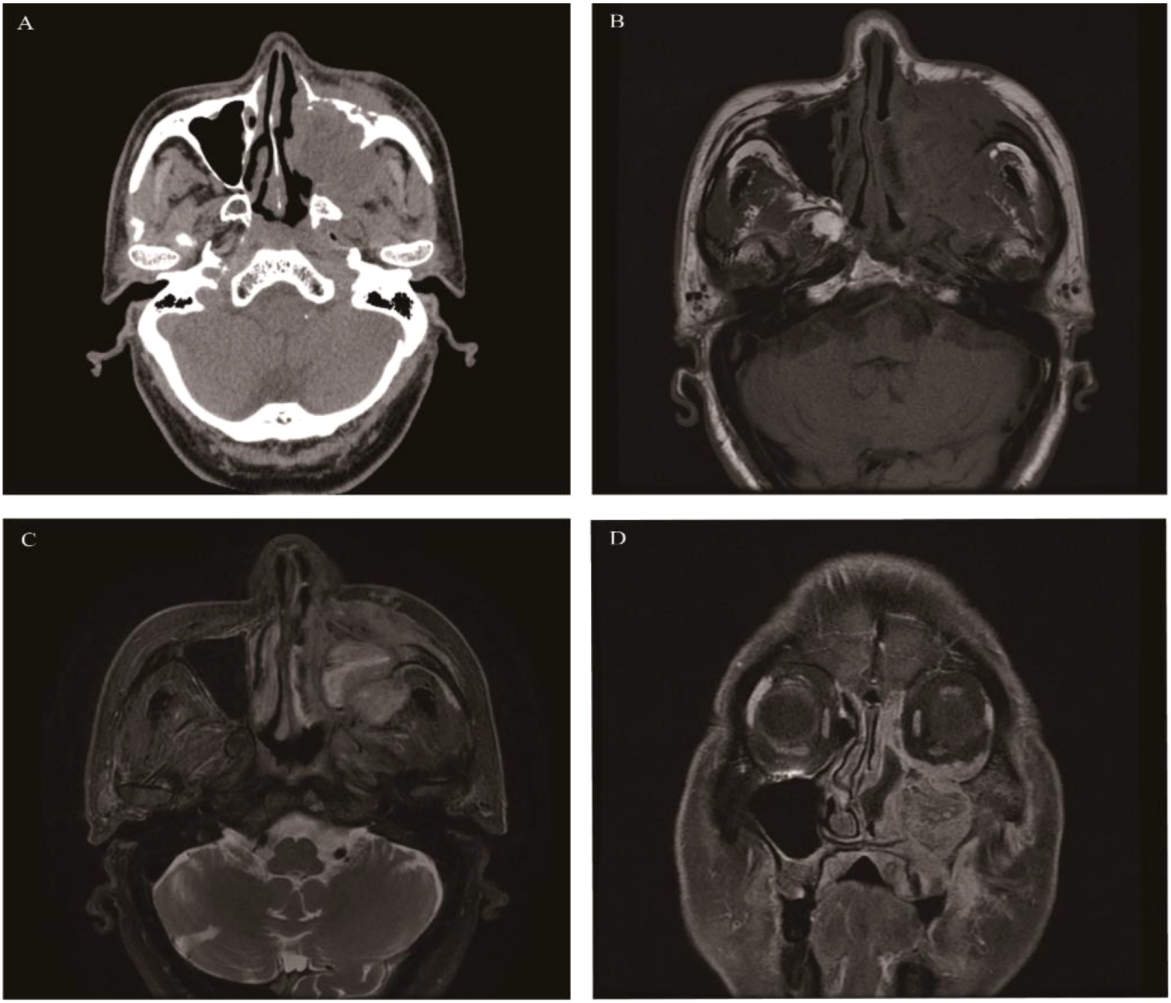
Adenoid cystic carcinoma: Axial CT revealed an irregular soft tissue mass in the left maxillary sinus with notable but less extensive bone destruction compared to SCC (A). On T1WI, the mass appeared isointense (B), while on T2WI (C), it exhibited a slightly hyperintense signal with strip- or tube-shaped hyperintense regions. Coronal contrast-enhanced T1WI displayed a heterogeneous “sieve-sac-like” pattern (D). Additionally, enhanced MRI indicated infiltration of the mass into the orbit, hard palate, and buccal mucosa.
